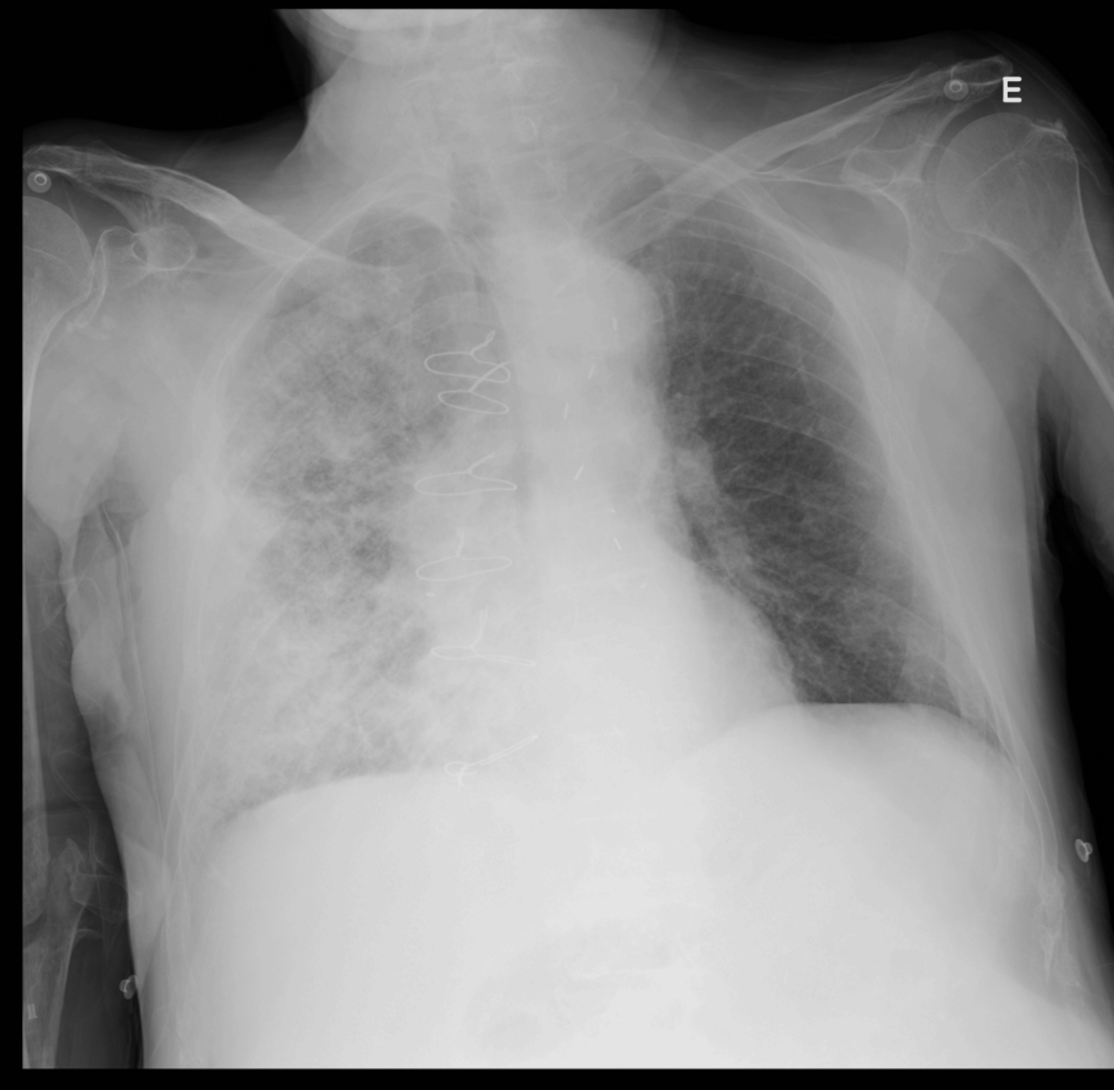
Chest X-ray (anteroposterior view) The chest X-ray revealed widespread opacities on the right side, more prominent in the middle and lower lung zones, suggesting consolidation. There was also a moderate pleural effusion on the right, evident by the blurring of the costophrenic angle and the silhouette of the right hemidiaphragm. The left lung appeared mostly clear. The heart size was within normal limits, and there were no signs of pneumothorax.
